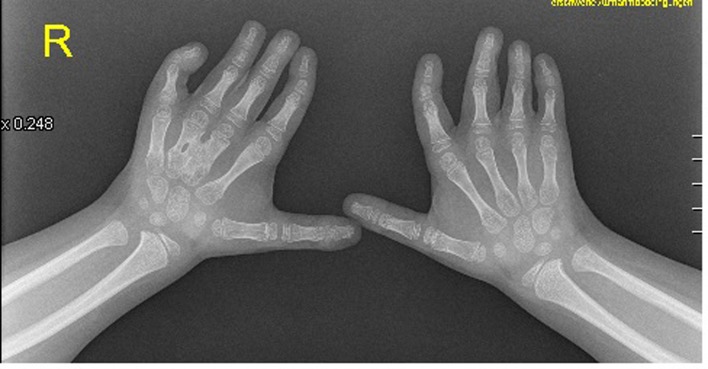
The hands were short with clinodactyly of the fifth fingers and his bilateral postaxial hexadactyly were operated early on. The feet were short with duplication of the hallux (polysyndactyly). Anteroposterior radiograph of the hands showed bilateral ulnar deviation, unilateral synostosis of the right third and fourth metacarpals, and marked dysplasia of the intermediate phalanges bilaterally.
